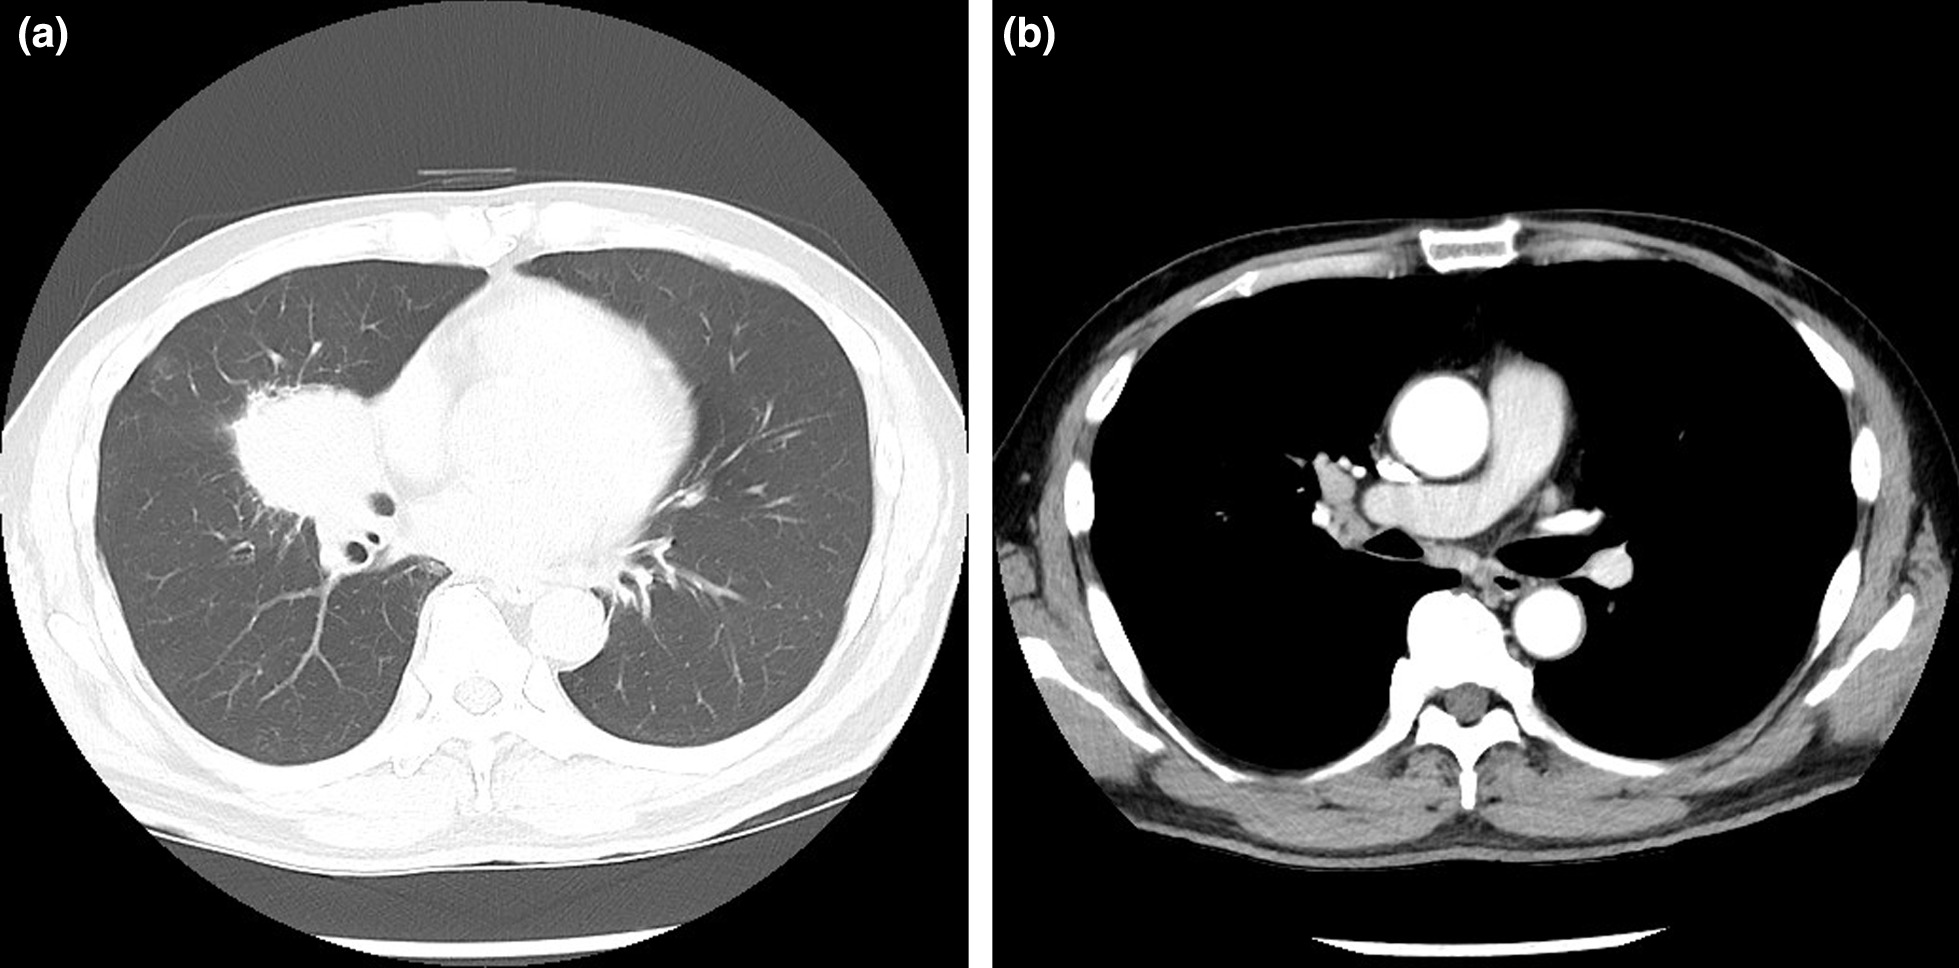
Chest CT. a, b Chest CT revealed a middle lobe hilar mass with irregular margins and swelling of the right hilar and mediastinal lymph nodes
